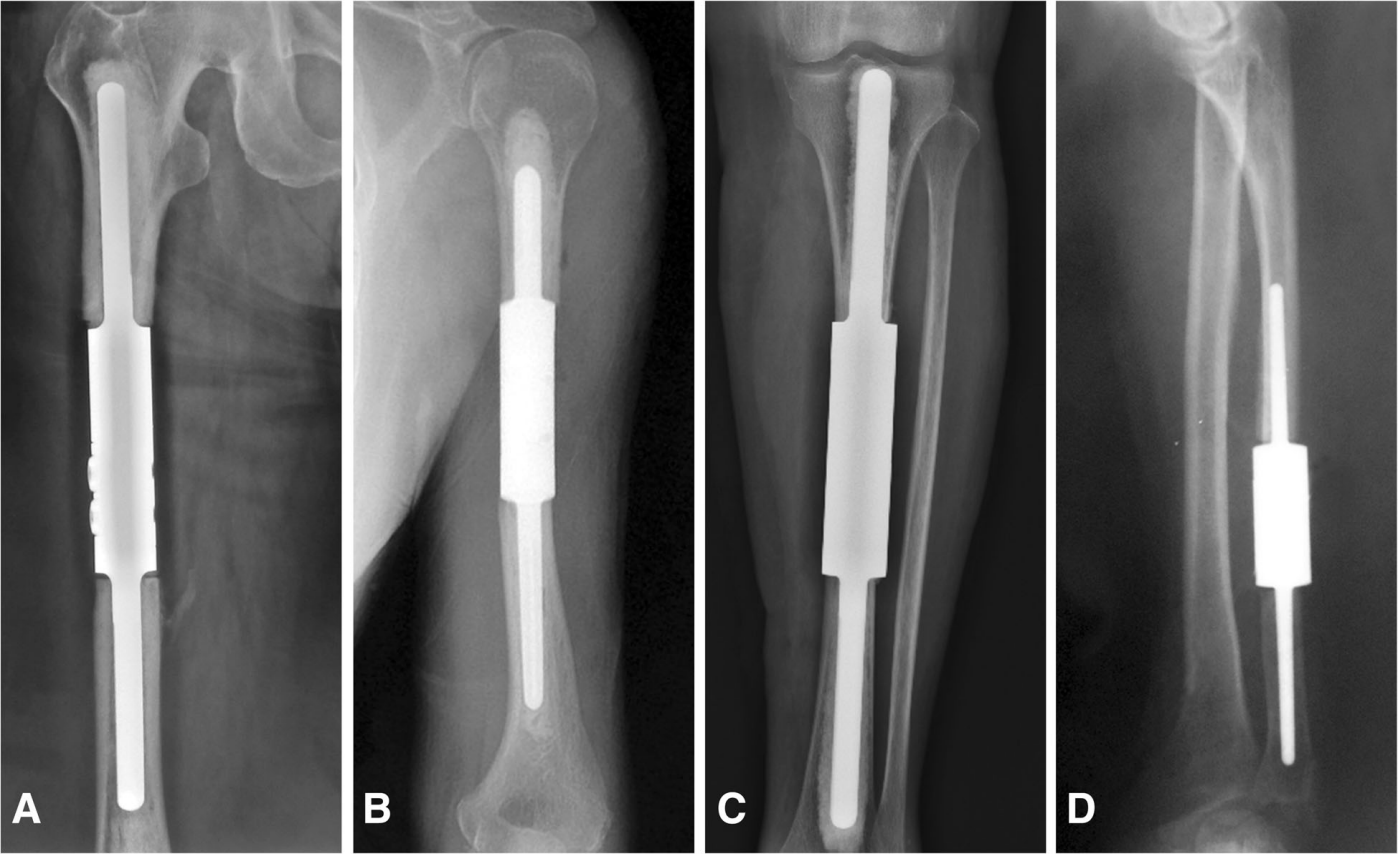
In this study, modular intramedullary segmental prosthesis reconstruction was performed in all patients, which included 30 (61.2%) femora, 13 (26.5%) humeri, five (10.2%) tibiae, and one (2.0%) ulna. Radiographs showing examples of diaphyseal reconstruction of the (a) femur, b humerus, c tibia, and d ulna using modular intramedullary segmental prostheses. For these four patients, no assistant plate had been used because the remaining bone marrow cavity was long enough
